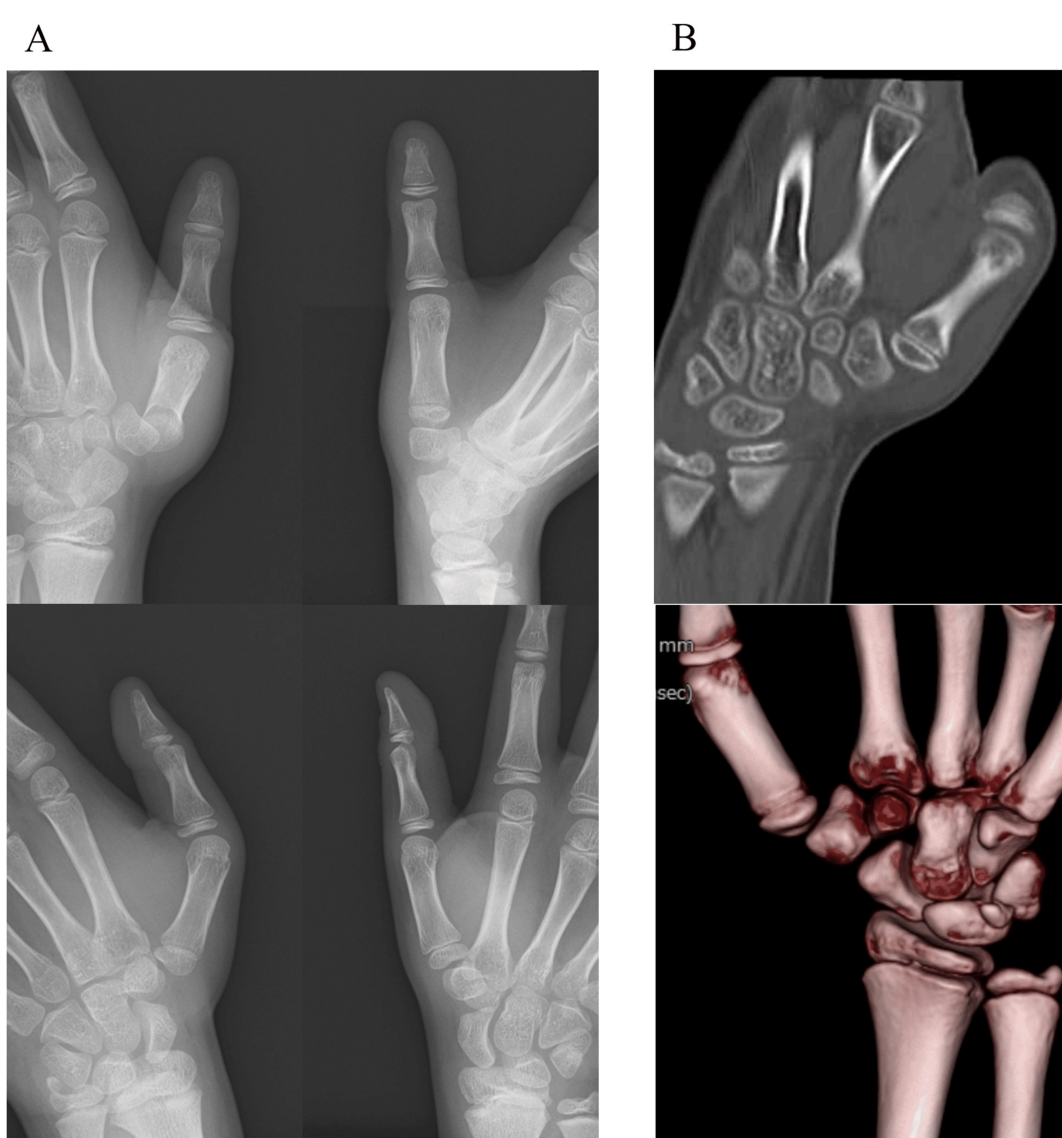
Images before the initial surgery. (A) Bilateral hand radiographs and (B) computed tomography images showing the dislocated left thumb carpometacarpal (CMC) joint without fractures.
